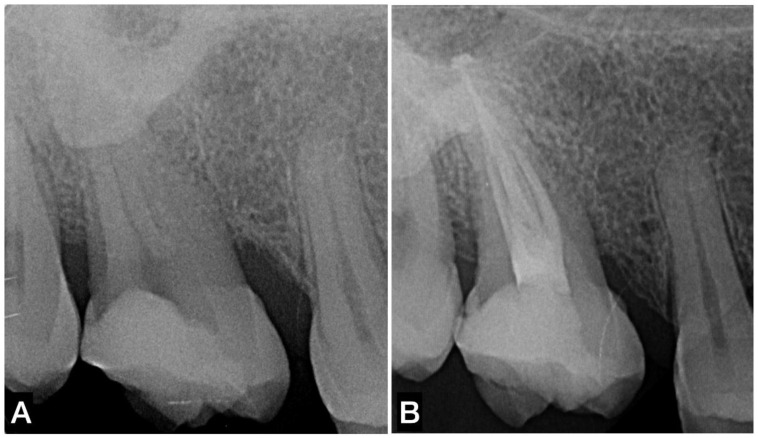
Example of indication of the single-cone technique with CSBS. (A) Preoperative periapical radiograph of a necrotic maxillary molar with long roots, sinus proximity, and patient’s limited mouth opening. (B) Postoperative periapical radiograph of root canal obturation using CSBS.
